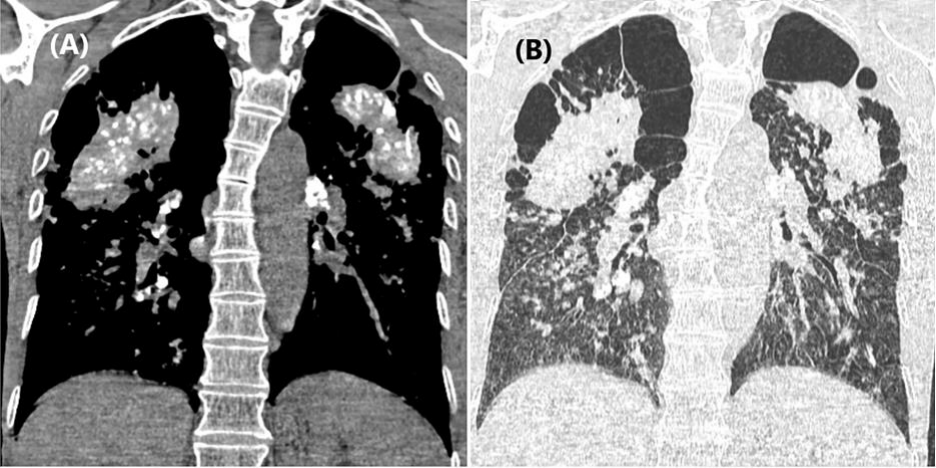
chest computed tomography scan in mediastinal (A) and parenchymal (B) windows on coronal view, revealing large bilateral peri-hilar fibrotic masses involving the upper lobes with massive bullae of emphysema predominant in the apices
